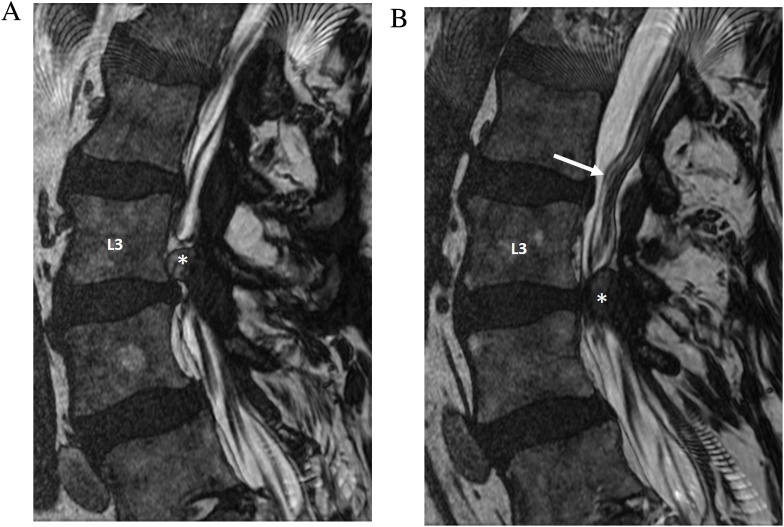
Spinal stenosis secondary to synovial cysts. A 75-year-old man who presented with radiculopathy. Sagittal FIESTA-C MRI images to the left (a) and to the right (b) of midline demonstrate bilateral synovial cysts (asterisks) which cause compression of the thecal sac and buckling of the cauda equina nerve roots (arrow).
